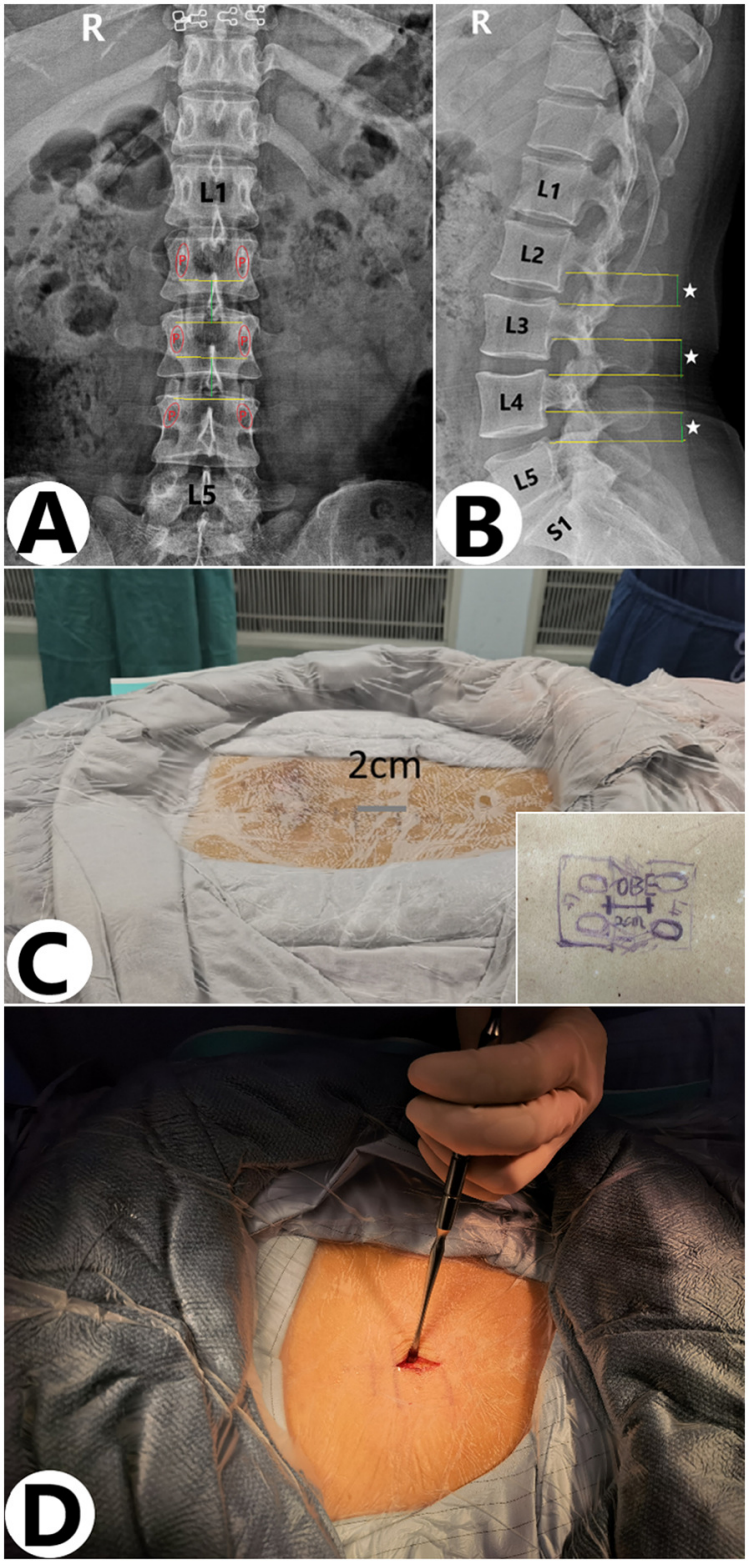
Surgical incision under x-ray guidance. (A,B) Under x-ray guidance, the incision extends from the lower edge of the superior vertebral arch to the upper edge of the inferior vertebral arch, forming a 2–3 cm posterior midline incision. (C,D) Actual size of the incision during surgery.
